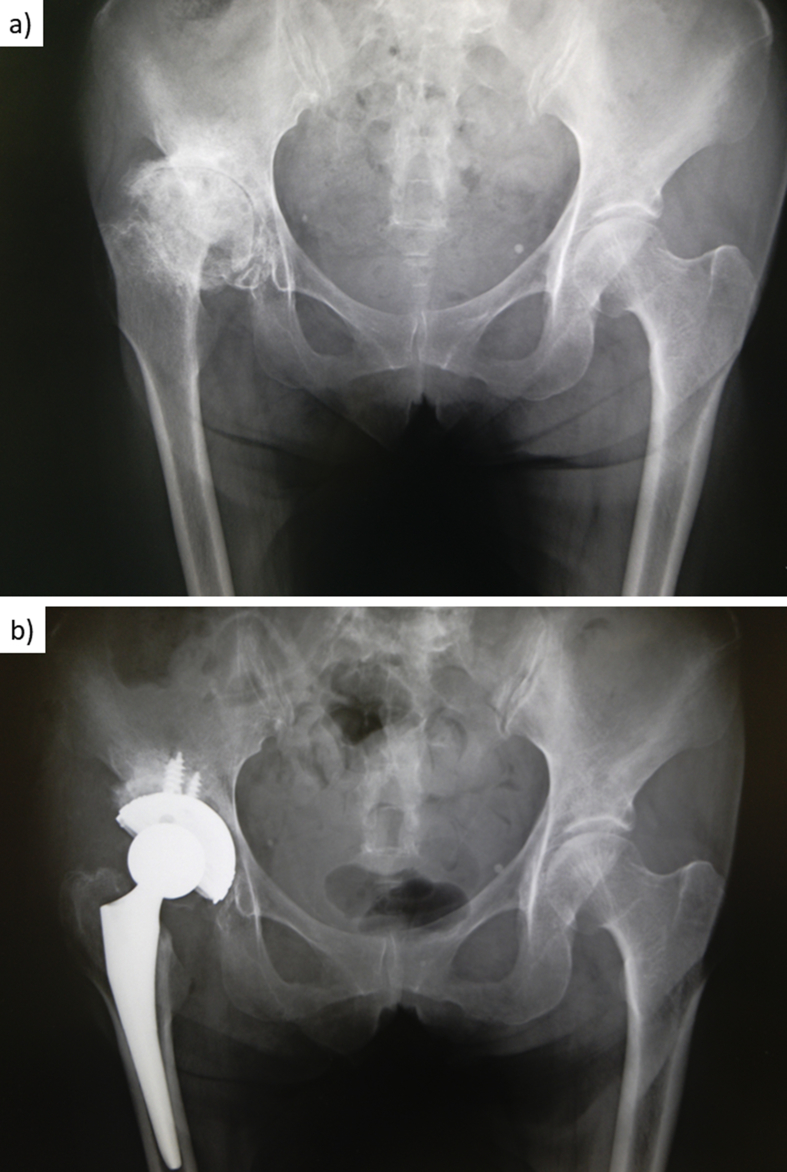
(a) Preoperative plain radiograph. The patient had developed coxarthrosis and severe pain of the right hip joint. Radiographs show stage 4 arthritis based on the Japanese Orthopaedic Association hip score. (b) Postoperative plain radiograph, which confirmed the implant's correct setting.
